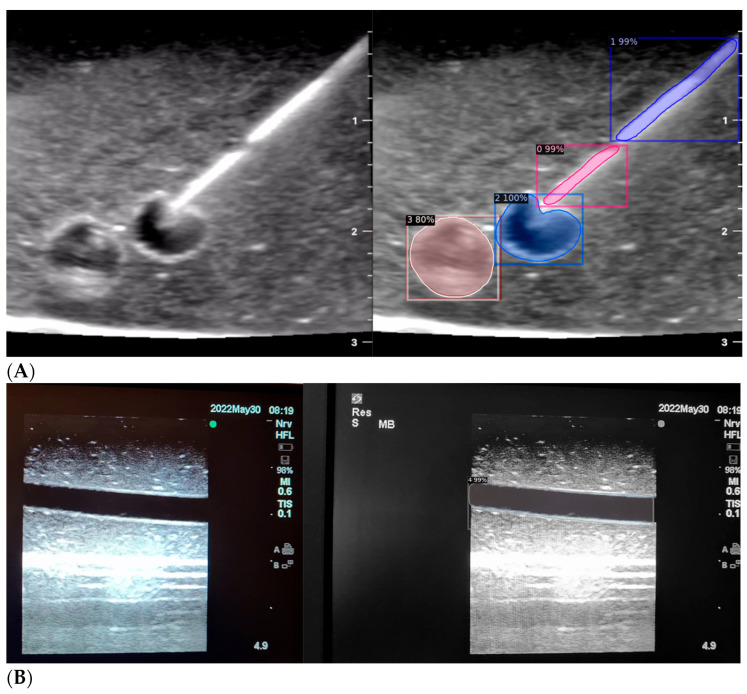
(A,B) AI-based program identifying needle tip (pink), needle shaft (purple), blood vessel (blue), and nerve (red) in ultrasound images.
